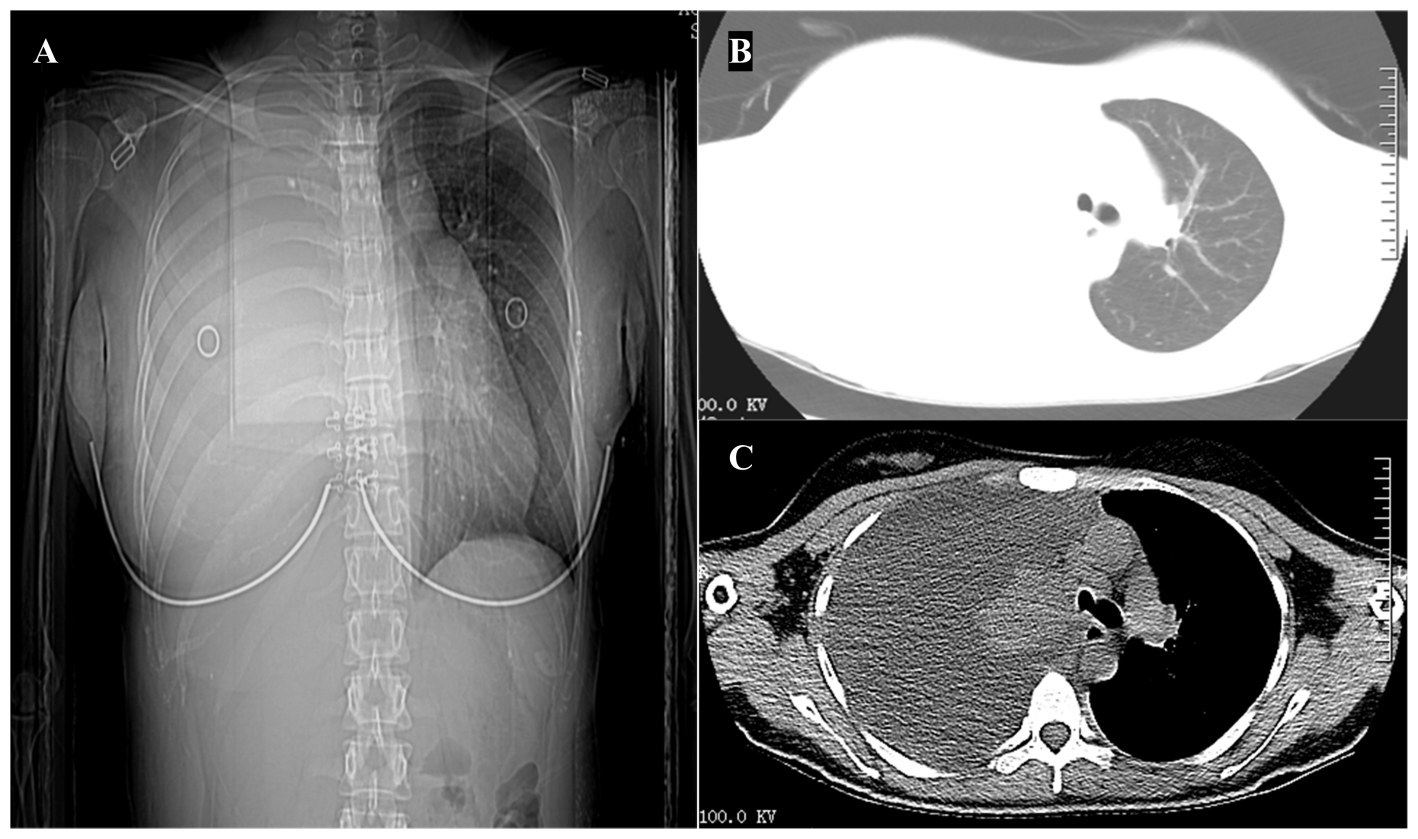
Imaging on hospitalization. (A) Chest x-ray demonstrated a right-sided pleural effusion. (B, C) Computed tomography scan of the chest showing a substantial amount of pleural effusion on the right side, with incomplete expansion of the right lung.
